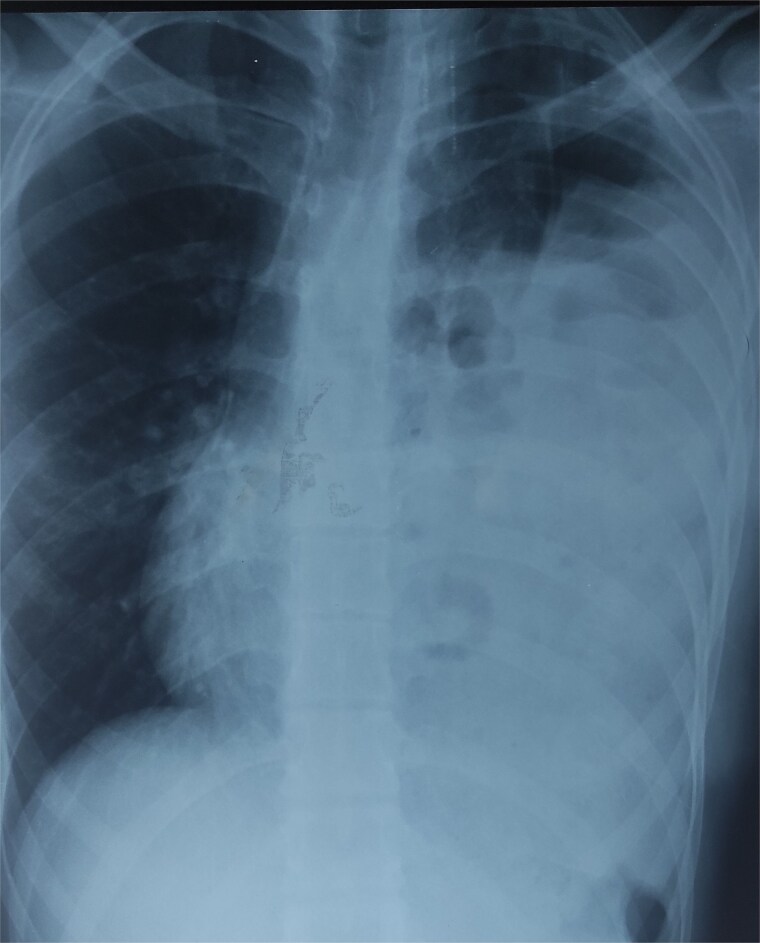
Chest X-ray showing air-filled bowel loops within the left hemithorax.
